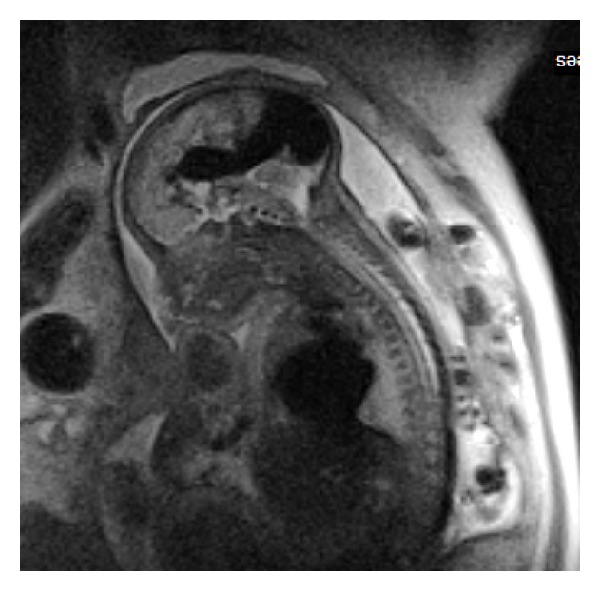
Fetal MRI—23rd week of gestation. SSFSE, T2WI, sagittal plane. Vein of Galen malformation (VOGM) causing callosal hypoplasia.
